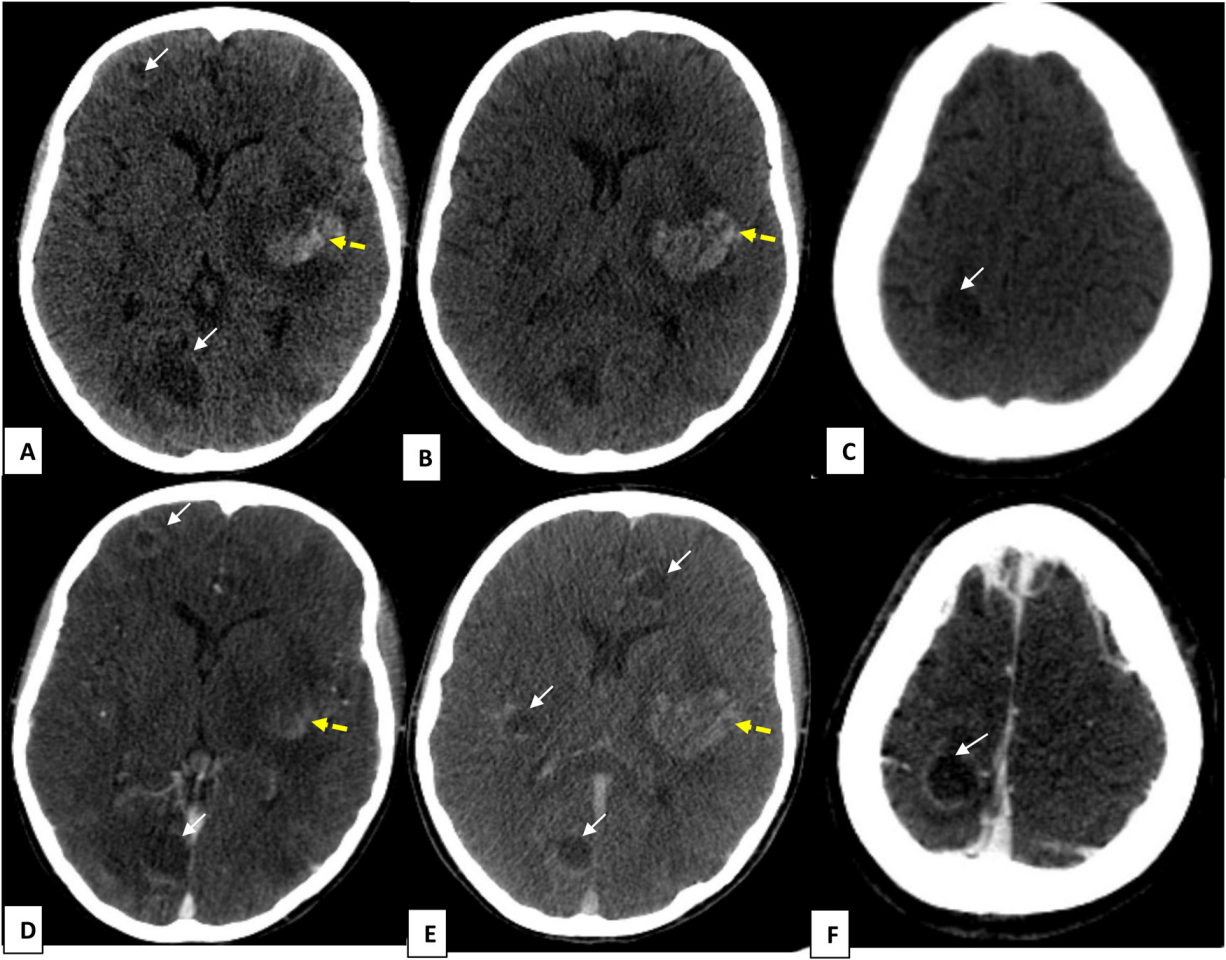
Brain CT scan before (A–C) and after contrast injection (D–F) shows multiple supratentorial cerebral lesions with fluid-filled centers and smoothly enhancing walls after contrast administration (white arrows), associated with a left capsulolenticular hematoma (yellow-dashed arrows), with associated surrounding edema.
